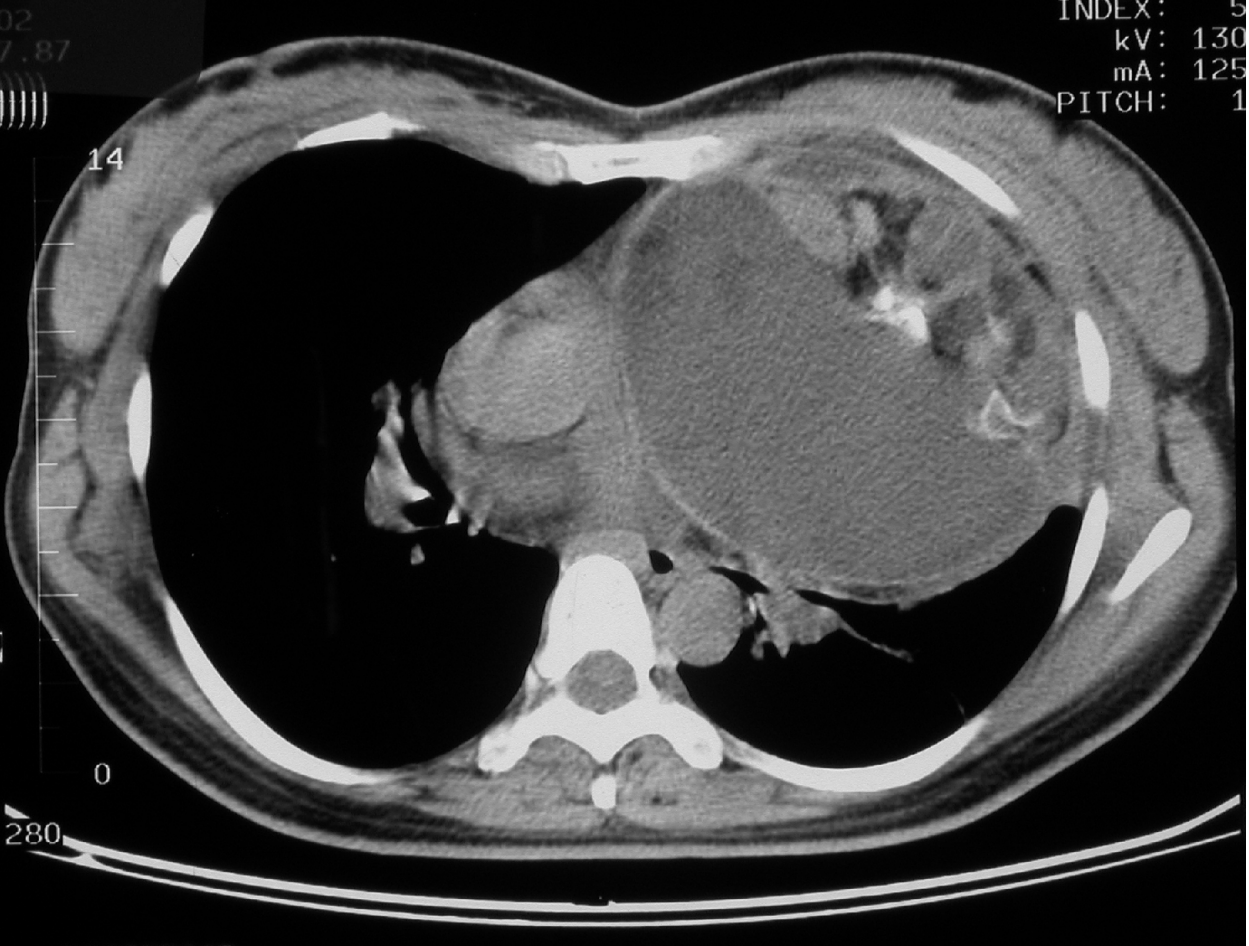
Preoperative CT-scan of the chest revealing the mass compressing the mediastinal vessels and airway.
